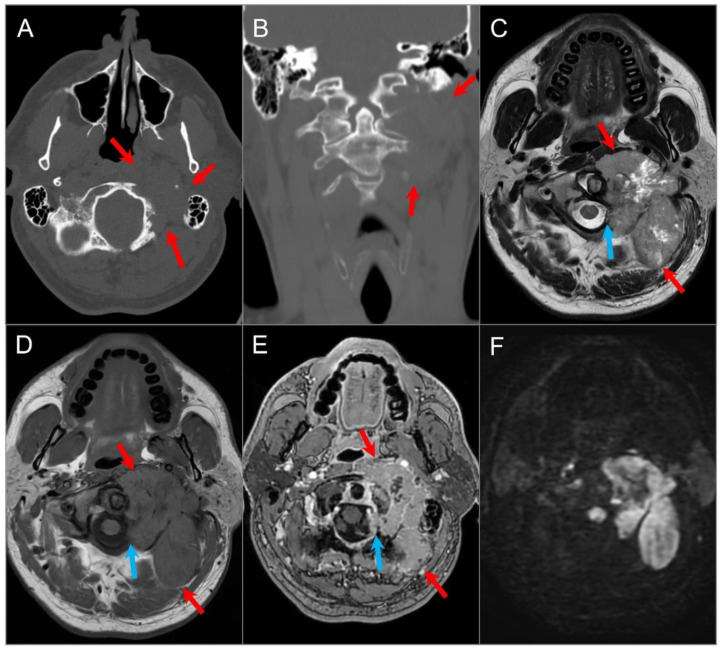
Skull base metastasis from RCC. (A) Axial and (B) coronal CT images show a lucent destructive soft tissue density mass lesion (red arrows) involving the left occipital bone, including occipital condyle, left lateral mass of C1, and inferomedial mastoid. (C) T2W, (D) noncontrast T1W, and (E) postcontrast T1W images reveal a bulky mass (red arrows) involving the left skull base and extending to the left retropharyngeal region and left epidural space (blue arrows). The lesion shows avid enhancement with central cystic/necrotic degeneration. (F) DWI image shows hyperintense signal in the solid components of the lesion consistent with restricted diffusion due to high cellularity.
